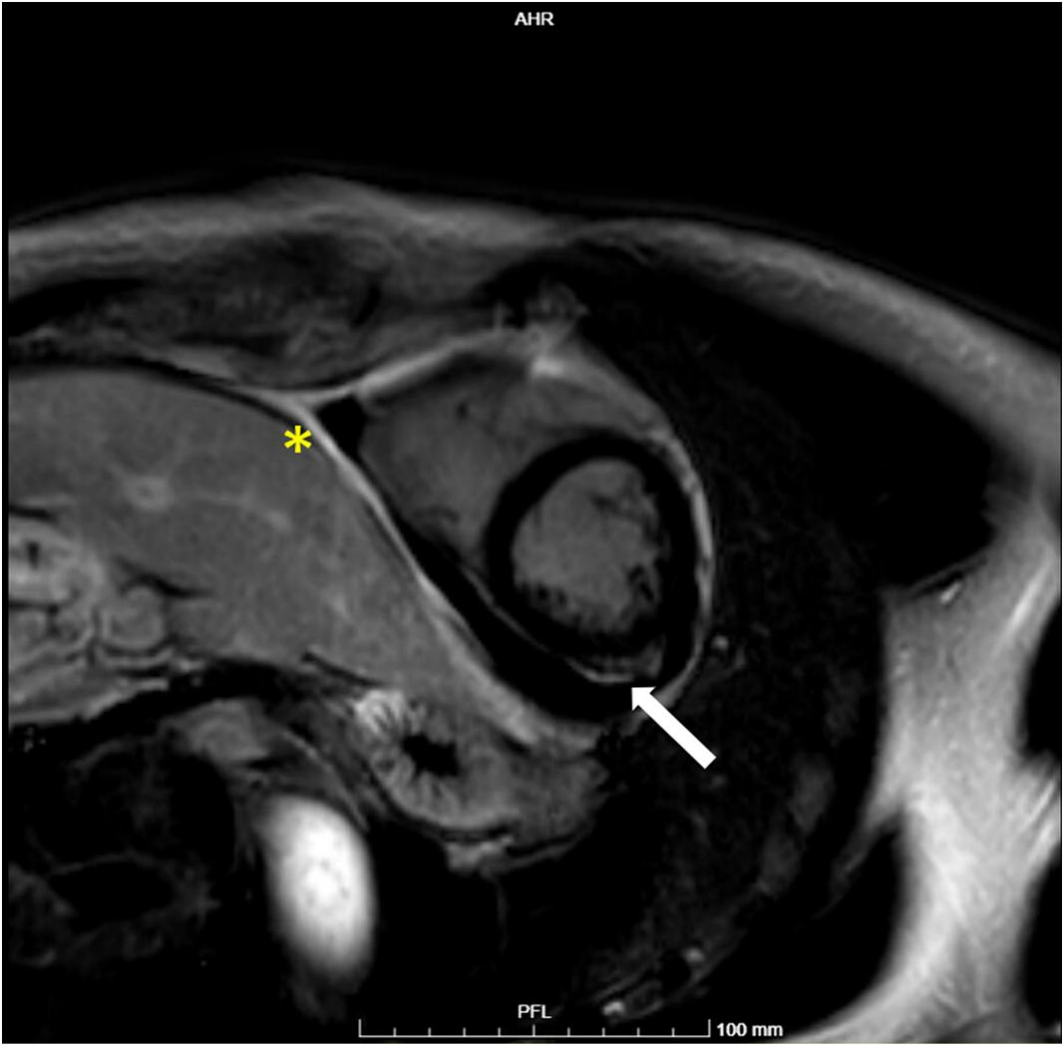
Cardiac MRI, T1-weighted sequence showing pericardial (asterisk) and a subepicardial area of LGE (arrow).
